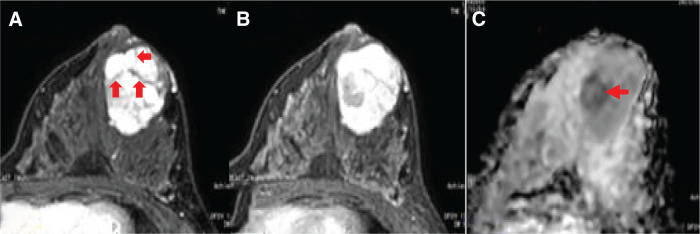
Dynamic MRI: (A) early phase. (B) Late phase. The tumor showed heterogeneous enhancement from the early phase, and contrast enhancement persisted in the late phase. Dark internal septa were observed inside (arrows). (C) Diffusion image: ADC map. Within the mass, a solid area of about 15 mm in diameter with diffusion-limited was observed (arrow).
